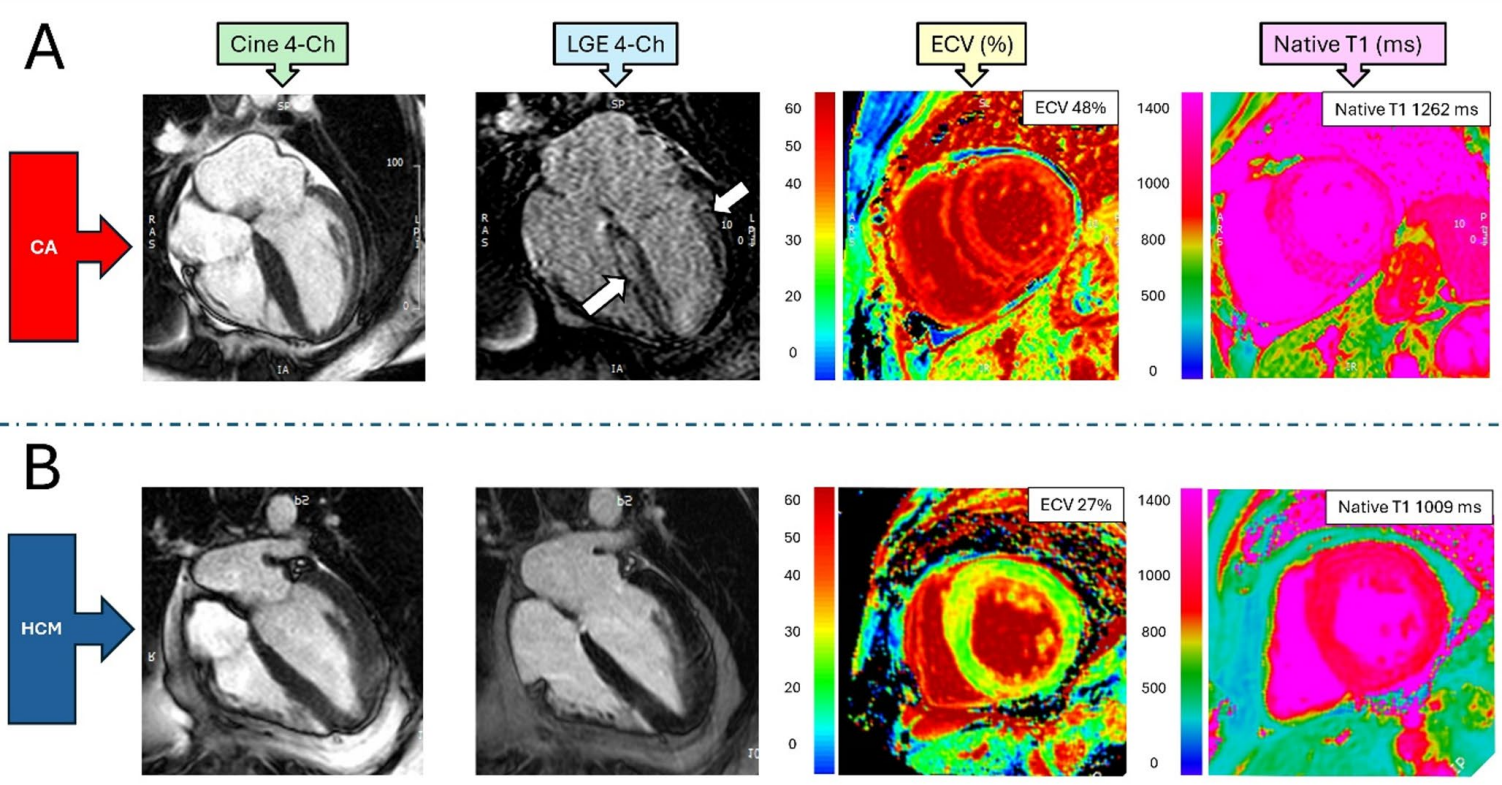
Representative patients who underwent multiparametric tissue characterization for undifferentiated LV hypertrophy. Patient (A) is 57 year old female. LGE sequences demonstrated diffuse subendocardial to midmyocardial delayed enhancement (white arrows) with an elevated ECV of 48% and elevated native T1 of 1262 ms at 1.5T cardiac MRI. This patient was subsequently diagnosed with AL amyloidosis. Patient (B) is a 53 year old male, LGE sequences demonstrated RV insertion point LGE (not shown) and a normal ECV of 27% and native T1 of 1009 ms. This patient was subsequently diagnosed with HCM. AL = amyloid light chain; 4-Ch = 4-chamber; CA = cardiac amyloidosis; ECV = extracellular volume fraction; HCM = hypertrophic cardiomyopathy; LGE = late gadolinium enhancement; LV = left ventricle; RV = right ventricle
